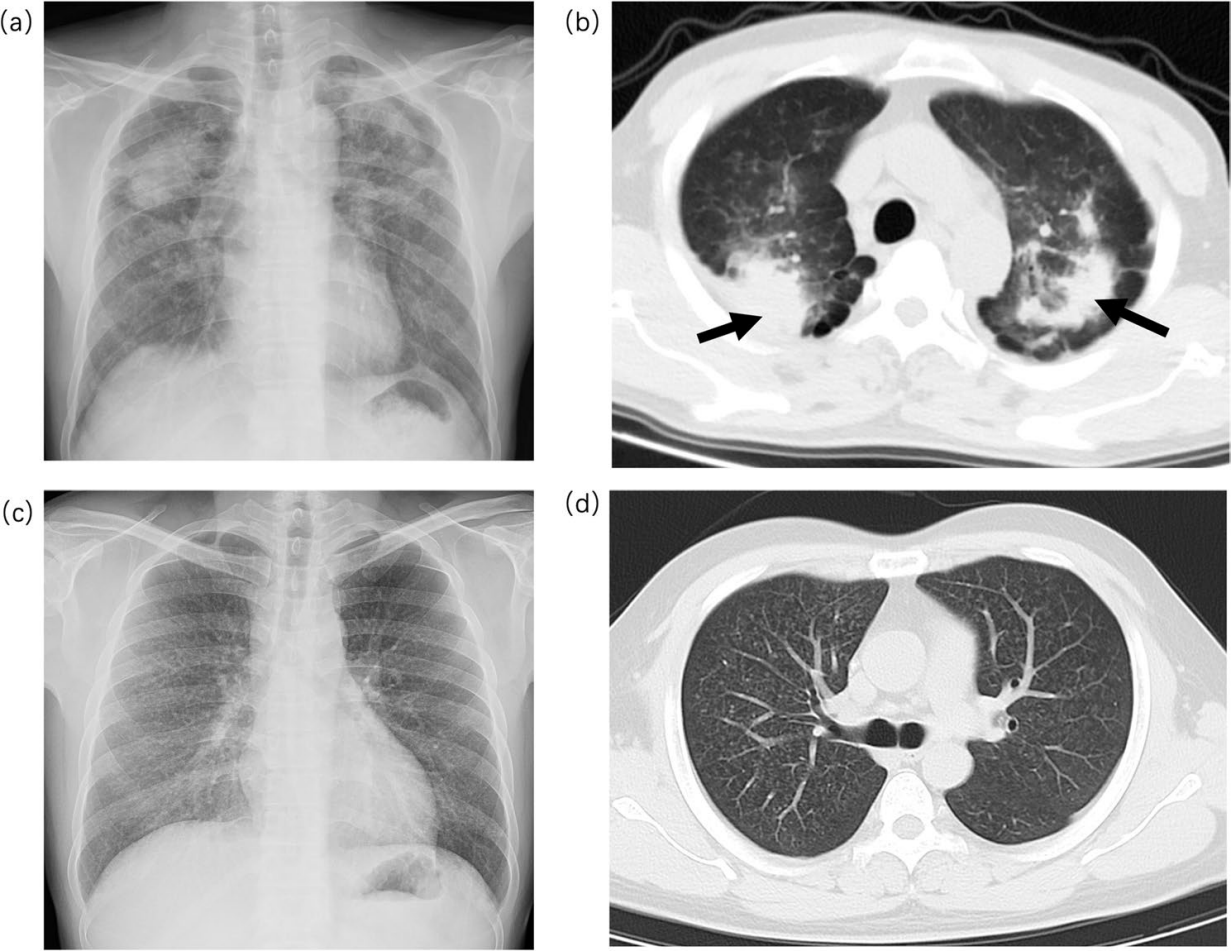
Typical imaging features of simple silicosis (CS) and complicated silicosis (SS). a, b Representative chest radiograph and high-resolution computed tomography images of CS, with the arrow indicating a progressive massive fibrosis lesion. c, d Representative chest radiograph and high-resolution computed tomography images of SS
